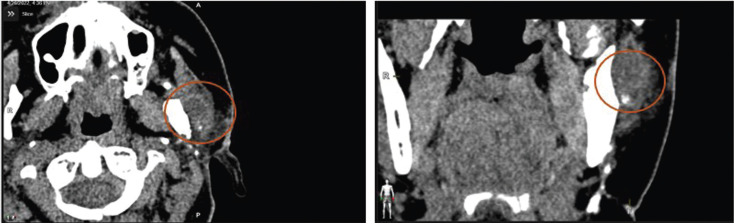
Preoperative CT scan. Red circle marks tumor lesion.
